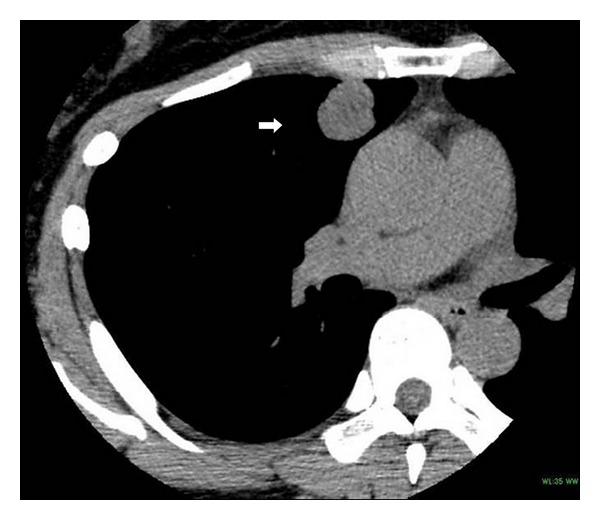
CT of the chest revealing a 2.0 × 2.0 cm nodular mass on the right peripheral upper lobe.
